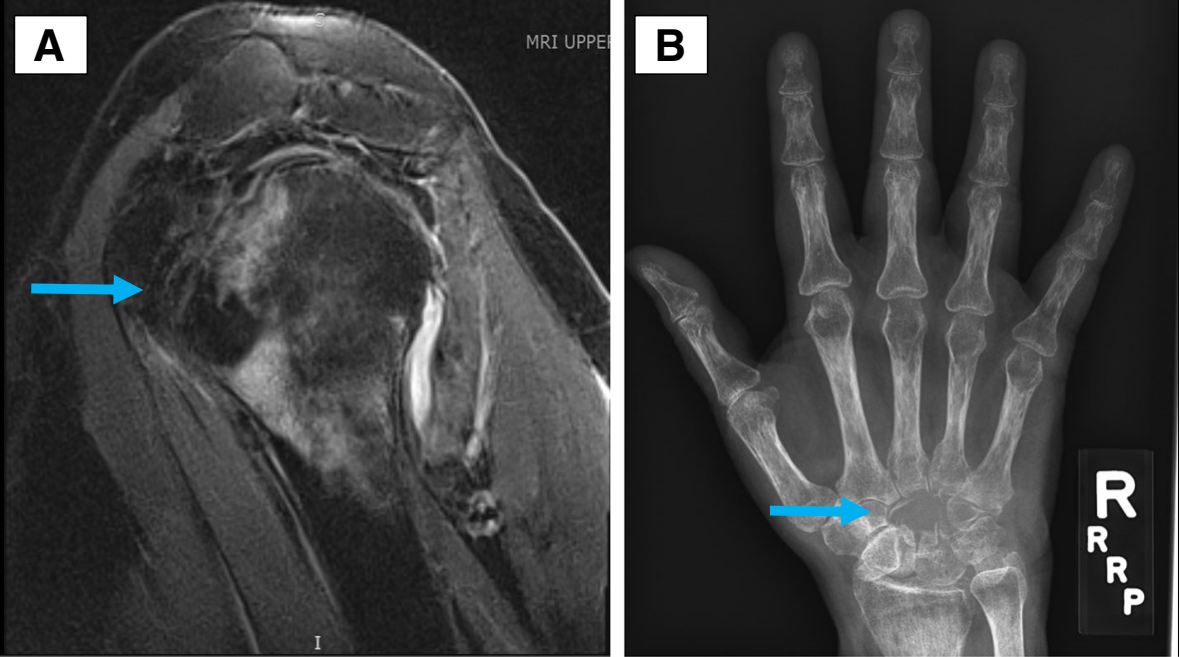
a Patient 4, MRI left shoulder with erosive changes of the glenohumeral articulation (arrow). b Patient 5, X-ray of the right hand with hamate and capitate resorption (arrow)
